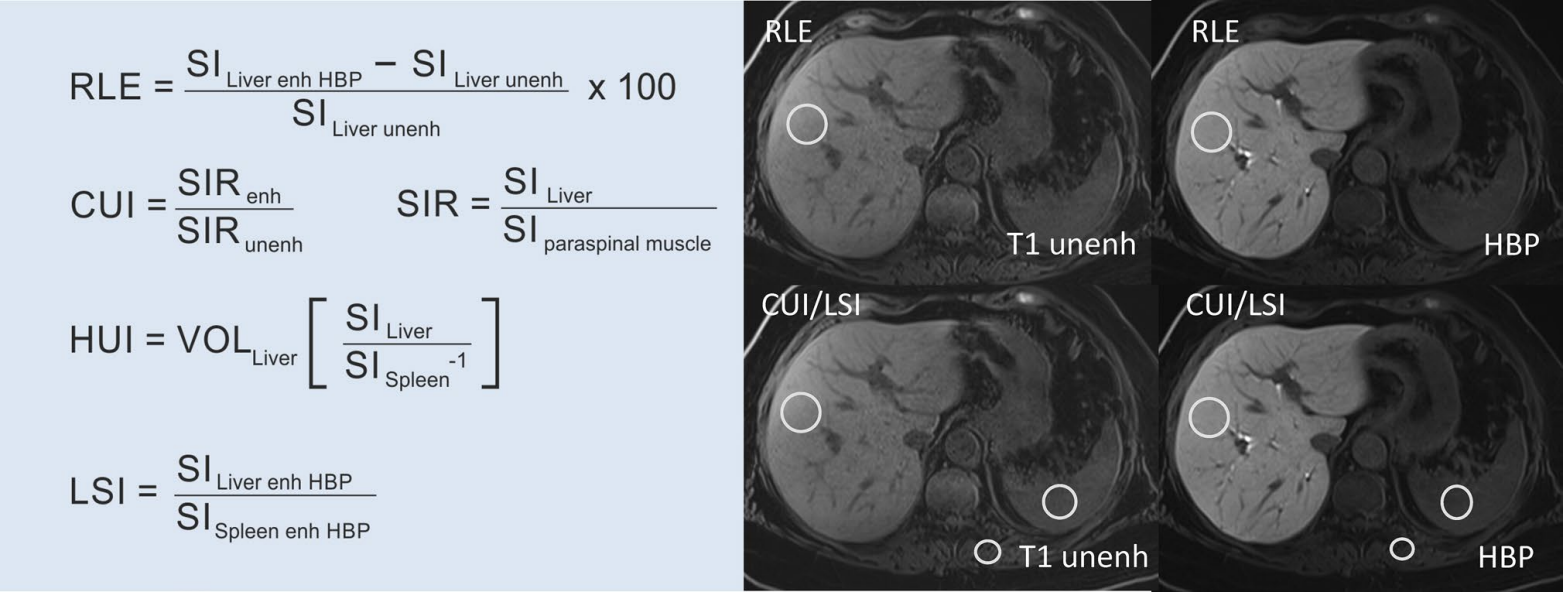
Equations for calculation of gadoxetic acid uptake for MRI scores. *RLE* relative liver enhancement, *CUI* contrast uptake index, *HUI* hepatic uptake index, *LSI* liver-spleen index, *SI* signal intensity, *SIR* signal intensity ratio, *enh* enhanced, *unenh* unenhanced
